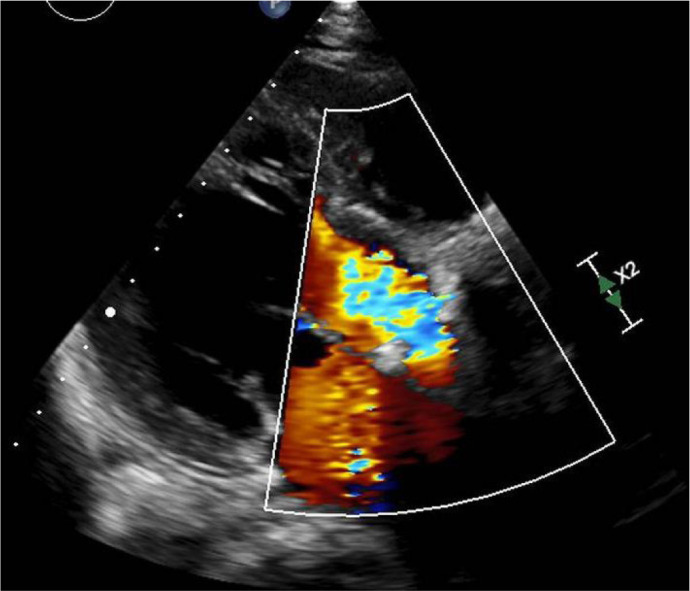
Transthoracic echocardiogram showing severe ventricular dilatation (left
